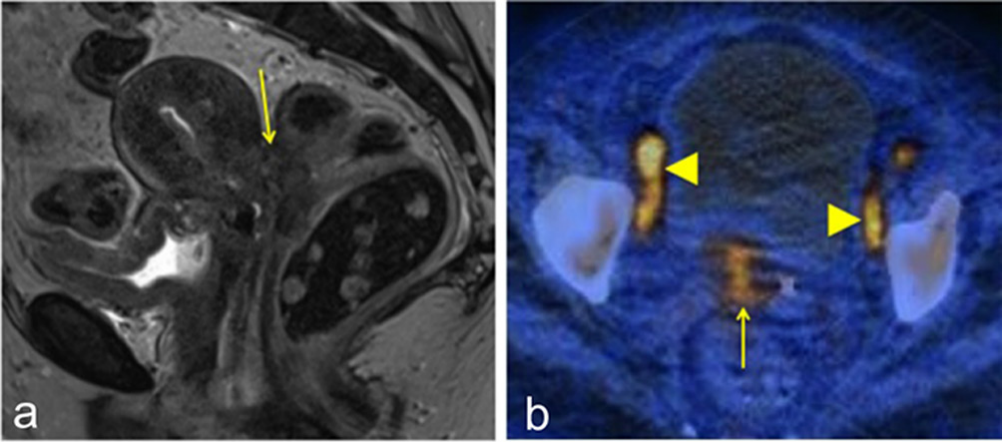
A 32-year-old female with SCC of the cervix, FIGO Stage IIb, following EBRT. (a) Sagittal T2-weighted image of the pelvis, demonstrates residual disease in the cervix, pouch of Douglas and rectal serosa (arrow). The MRI appearances suggested suitability for pelvic exenteration, and ^18^F-FDG PET CT was performed for confirmation. (b) ^18^F-FDG PET CT demonstrates FDG avid bilateral subcentimetre external iliac nodes excluding exenteration. The right-sided FDG avid node was biopsied and confirmed metastatic SCC. EBRT,external beam radiotherapy; FDG, fluoro-2-deoxy-D-glucose; ^18^F-FDG PET CT, [fluorine-18]-fluoro-2-deoxy-D-glucose positron emission tomography; SCC, squamous cell carcinoma.
